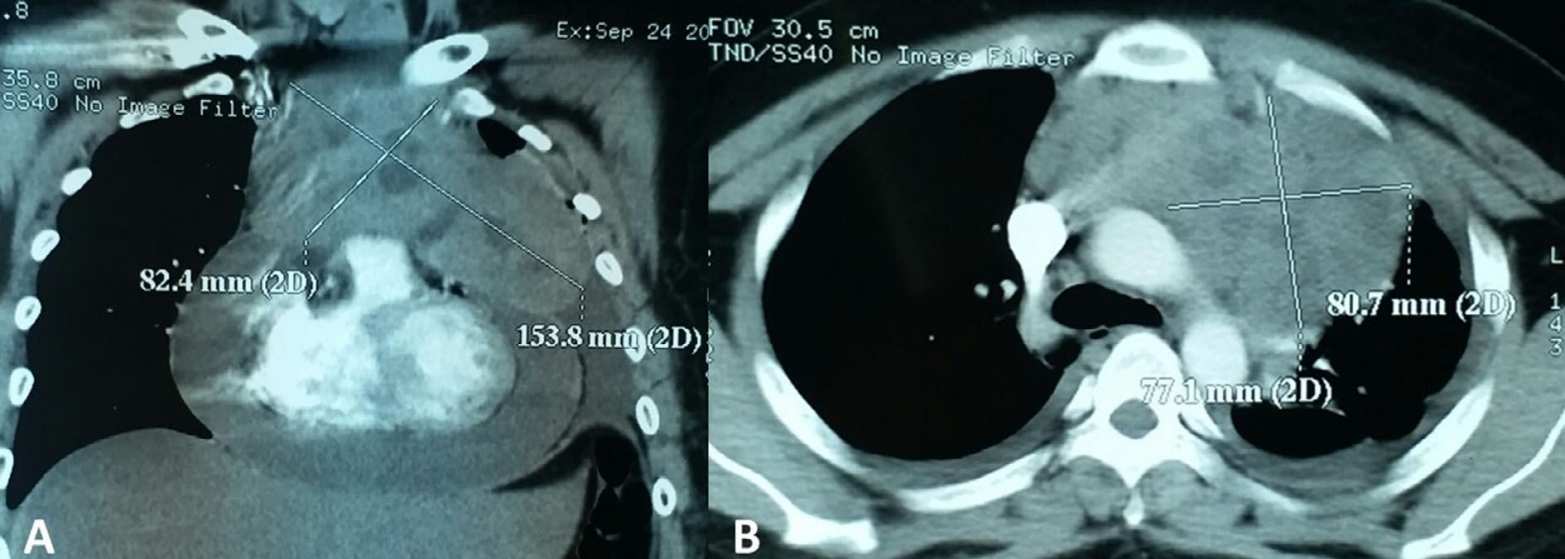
Mediastinal computed tomography (CT) scan showing the tumour. (A) Coronal image. (B) Axial image.
